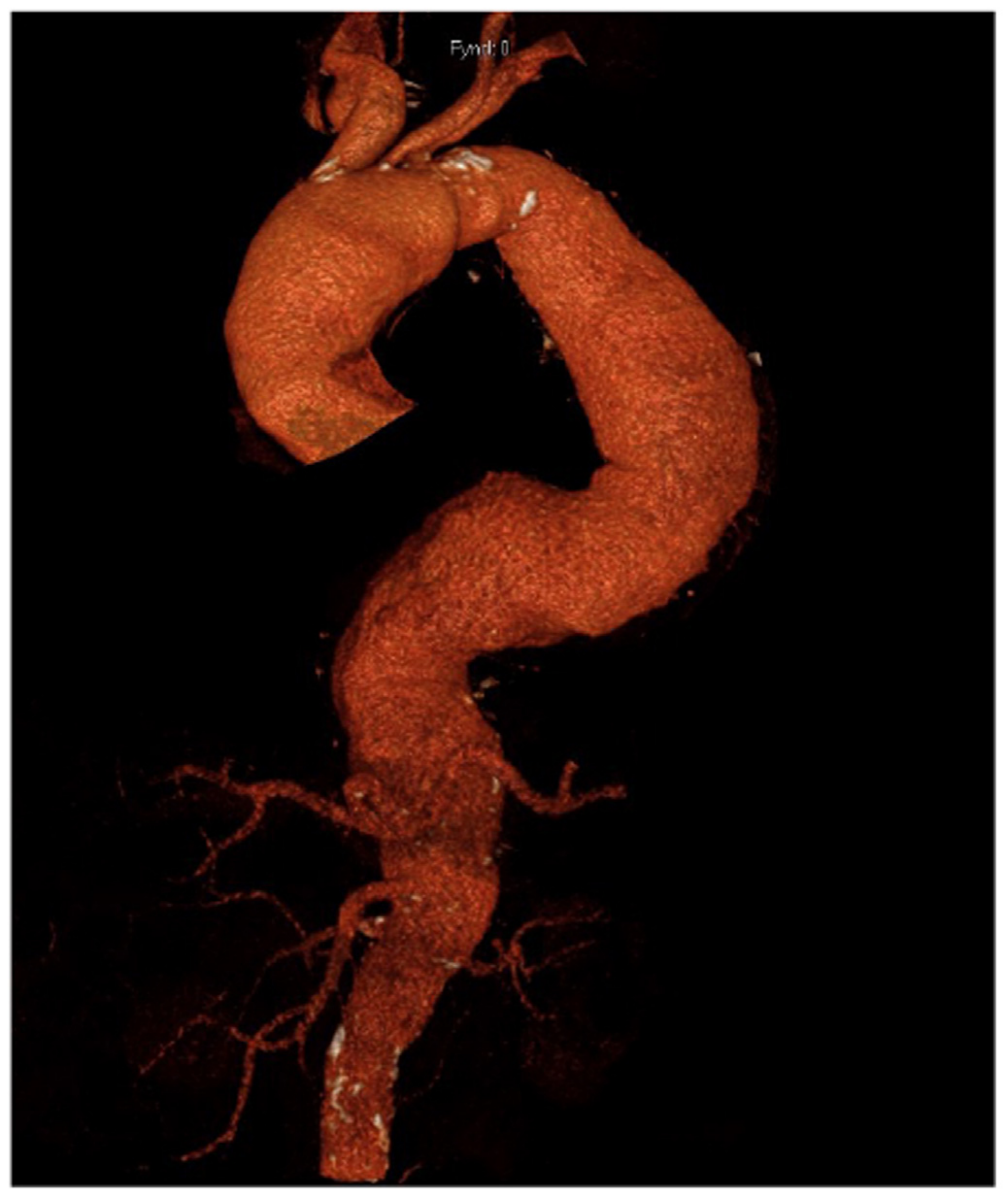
Three-dimensional reconstruction of preoperative computed tomography angiogram showing a Crawford type II aortic aneurysm.
